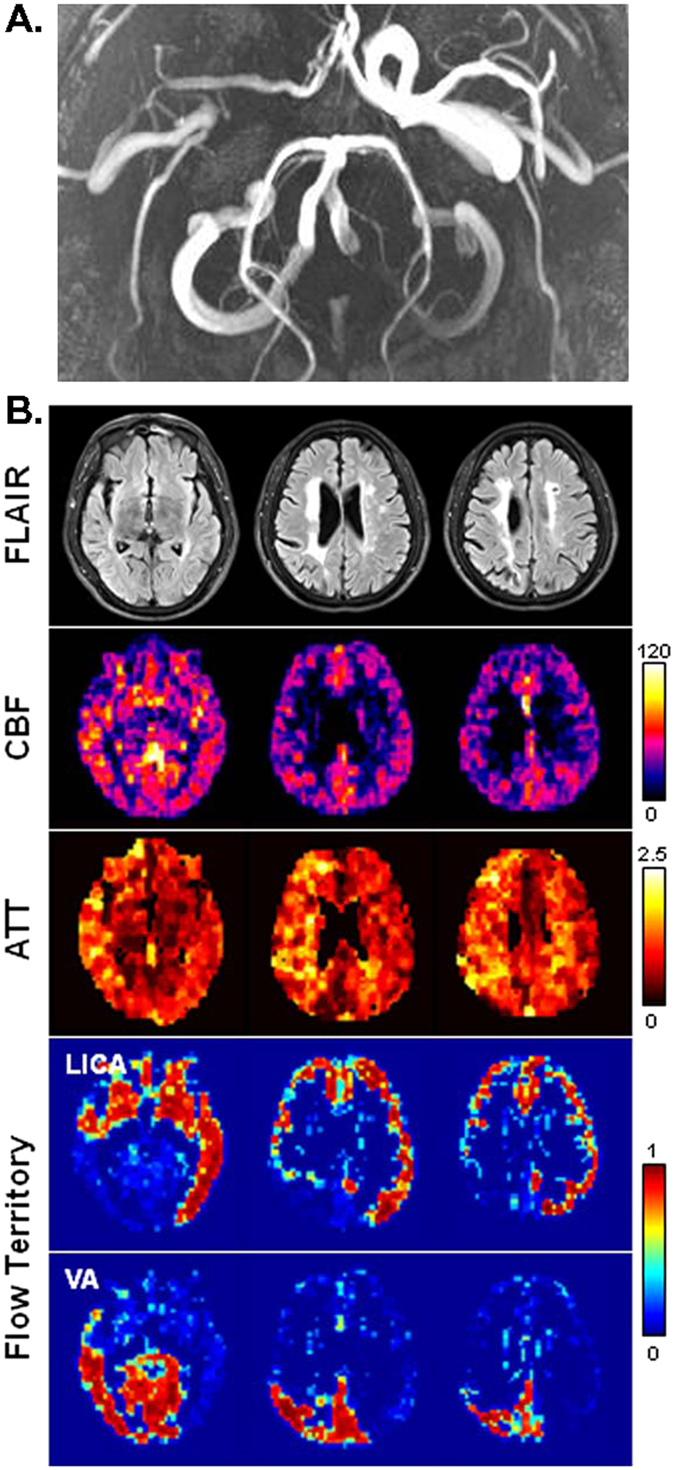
MR images obtained from a 57-year-old male patient with asymptomatic ICA steno-occlusive disease (>95% stenosis in the right ICA and 10–30% stenosis in the left ICA). (A) Time-of-flight angiography. Projection view from below. (B) From top to bottom rows (3 slices are shown in radiological convention): FLAIR images, CBF maps (in units of ml-blood/100 ml-tissue/min), ATT maps (in units of sec), and flow territory maps. Flow territory maps show the membership levels generated by fuzzy clustering, which range from 0 to 1. No contribution from right ICA was extractable. In FLAIR images, there are bilateral confluent periventricular white matter hyperintensities which are larger on the right side.
